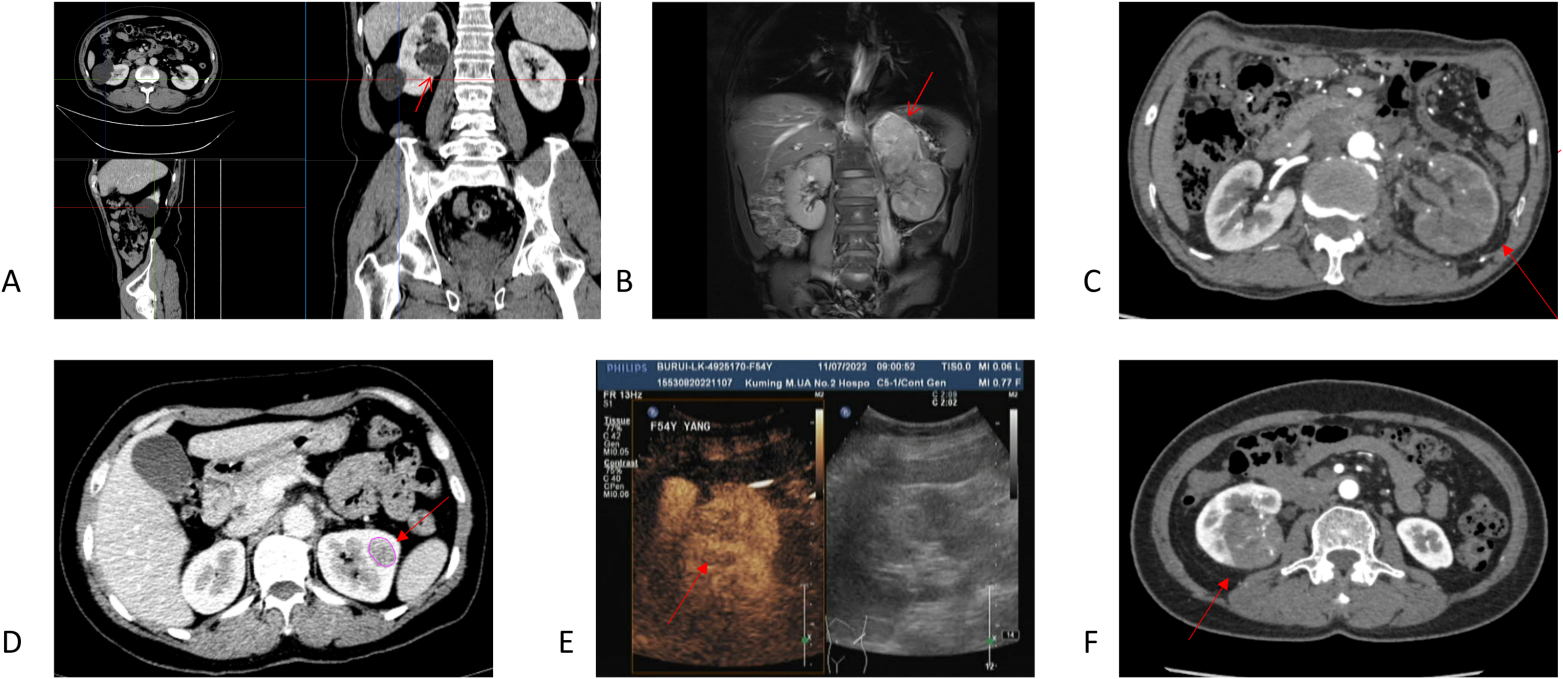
Imaging findings for Cases 1–4 [(A), Case 1; (B) and C. Case 2; (D, E), Case 3; F, Case 4]. (A) In Case 1, no enhancement was observed in the cystic region post-contrast. MPR view as shown, with arrows indicating the location of the suspected solid mass. (B, C) Case 2 demonstrates a T1- and T2-hyperintense mass in the left adrenal region, suggestive of adrenal metastasis. Renal artery CTA reveals no significant enhancement around the cystic lesion with sparse vascularity. (D, E) Case 3: A slightly hyperdense nodule with diminished enhancement in both parenchymal and delayed phases. Contrast-enhanced ultrasound imaging: The lesion demonstrated near-parenchymal enhancement in the early phase, with slow resolution in the late phase, appearing slightly hypointense. (F) Case 4: Non-contrast CT shows a well-defined tumor margin with a cystic–solid mixture. Contrast enhancement exhibits rapid uptake and washout, revealing punctate calcifications and patchy areas of increased density within the lesion.
